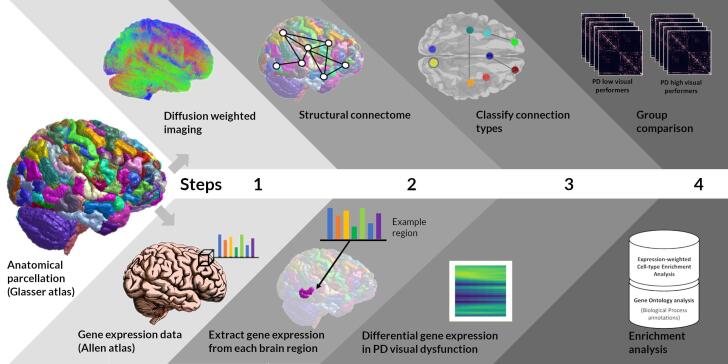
Overview of the study methodology. A1. Anatomically constrained tractography was used to determine white matter streamlines from diffusion weighted imaging (DWI) data for each participant. A2. DWI data were combined with anatomical parcellation of 379 brain regions using the Glasser atlas to generate a connectivity matrix for each participant. A3. Connection types were classified into interhemispheric (between hemispheres), intrahemispheric (within a hemisphere), intramodular (within a cortical module) and subcortial-cortical (between the subcortical regions and a cortical module). A4. Connection strength in different connection types was compared between PD low visual performers and PD high visual performers. B1. Gene expression data were extracted from the Allen Brain atlas and genes passing threshold expression were chosen. B2. Allen atlas samples were mapped into the 180 cortical regions from the left hemisphere according to the anatomical parcellation and an average cortical regional gene expression was calculated for each gene. B3. Partial least squares regression was performed for interhemispheric and basal ganglia-cortical connections in PD low visual performers. The regional gene expression in PD low visual performers associated with interhemispheric and subcortical-cortical white matter loss was calculated based on normalised gene weightings of the second PLS component. B4. Enrichment analysis was performed for the downweighted and upweighted genes that were significantly associated with connection loss for both connection types. Enrichment analysis included Gene Ontology (GO) biological processes, Expression-weighted Cell-type Enrichment analysis (EWCE) and enrichment for specific gene lists for PD, Alzheimer’s, Dementia with Lewy Bodies.
